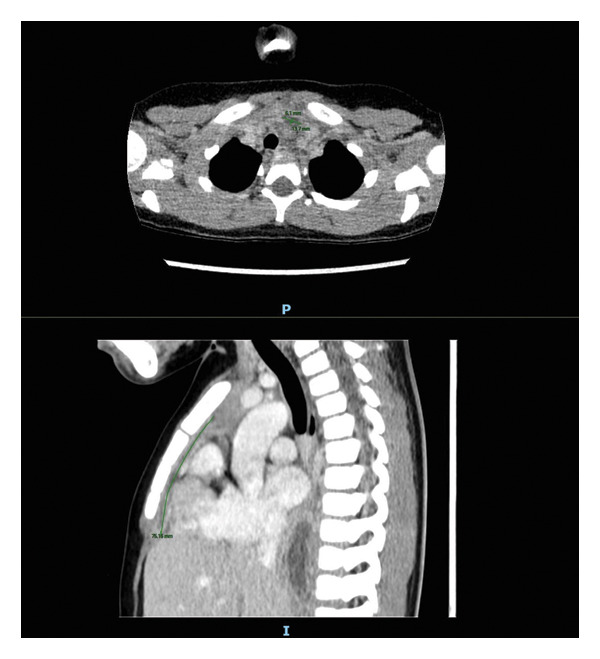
CT image from Case 1 showing the mediastinal fluid collection. The superimposed green lines indicate its maximum long‐ and short‐axis dimensions. P, posterior; I, inferior.
